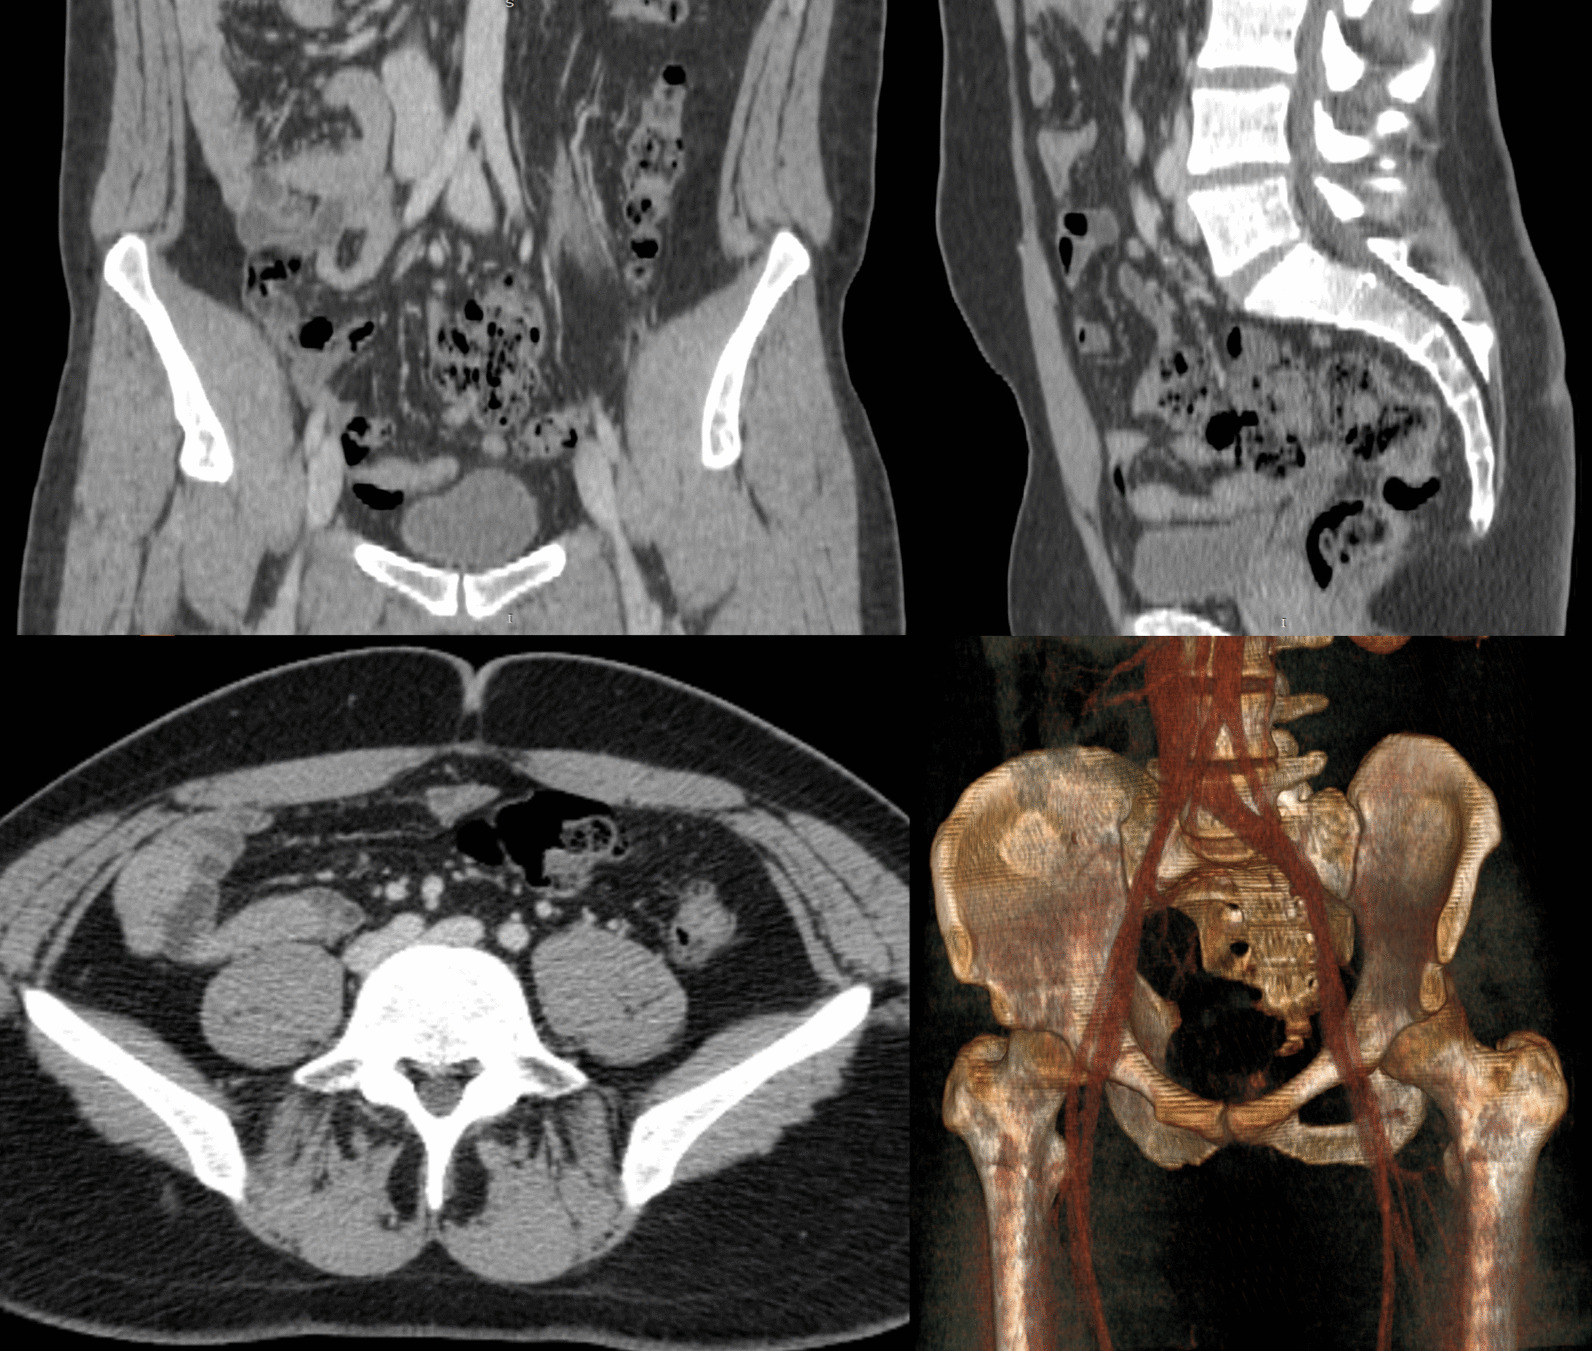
CT scans in all planes with 3D rendering demonstrate severe compression of the left common iliac vein by the right common iliac artery. 3D rendering demonstrates MTS-associated changes of the vein underlying the artery
